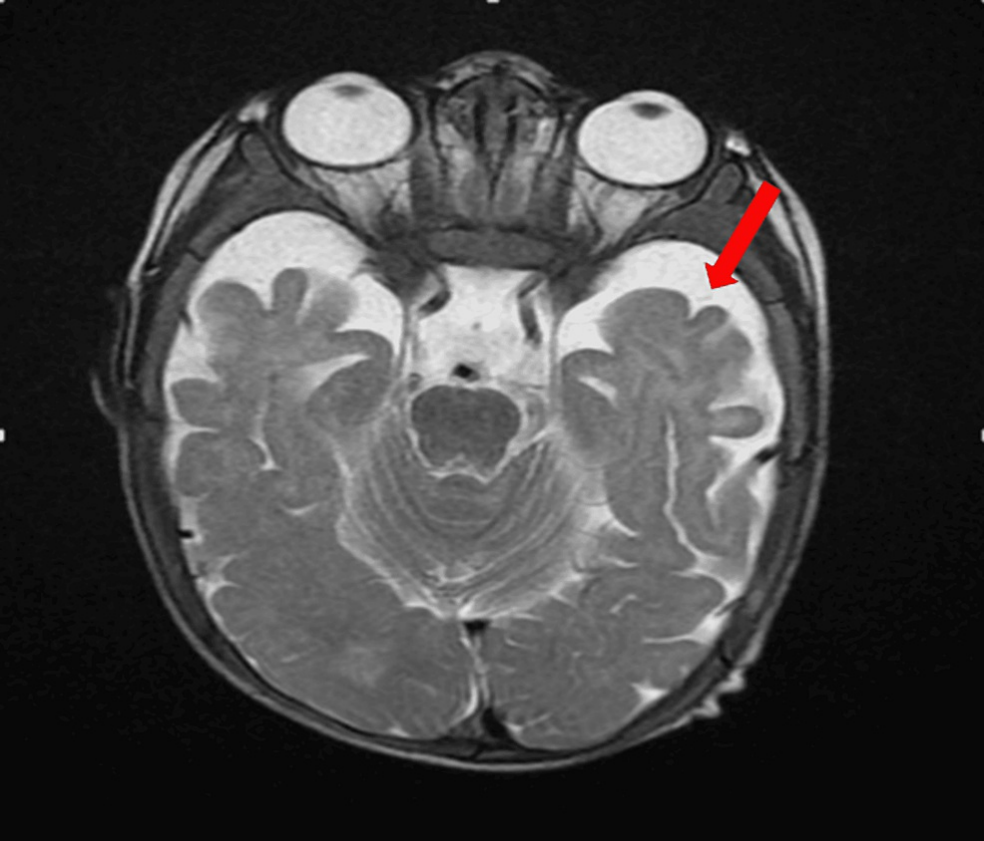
Axial section of a brain MRI scan showing widening of the cortical sulci in relation to cortical atrophy.
